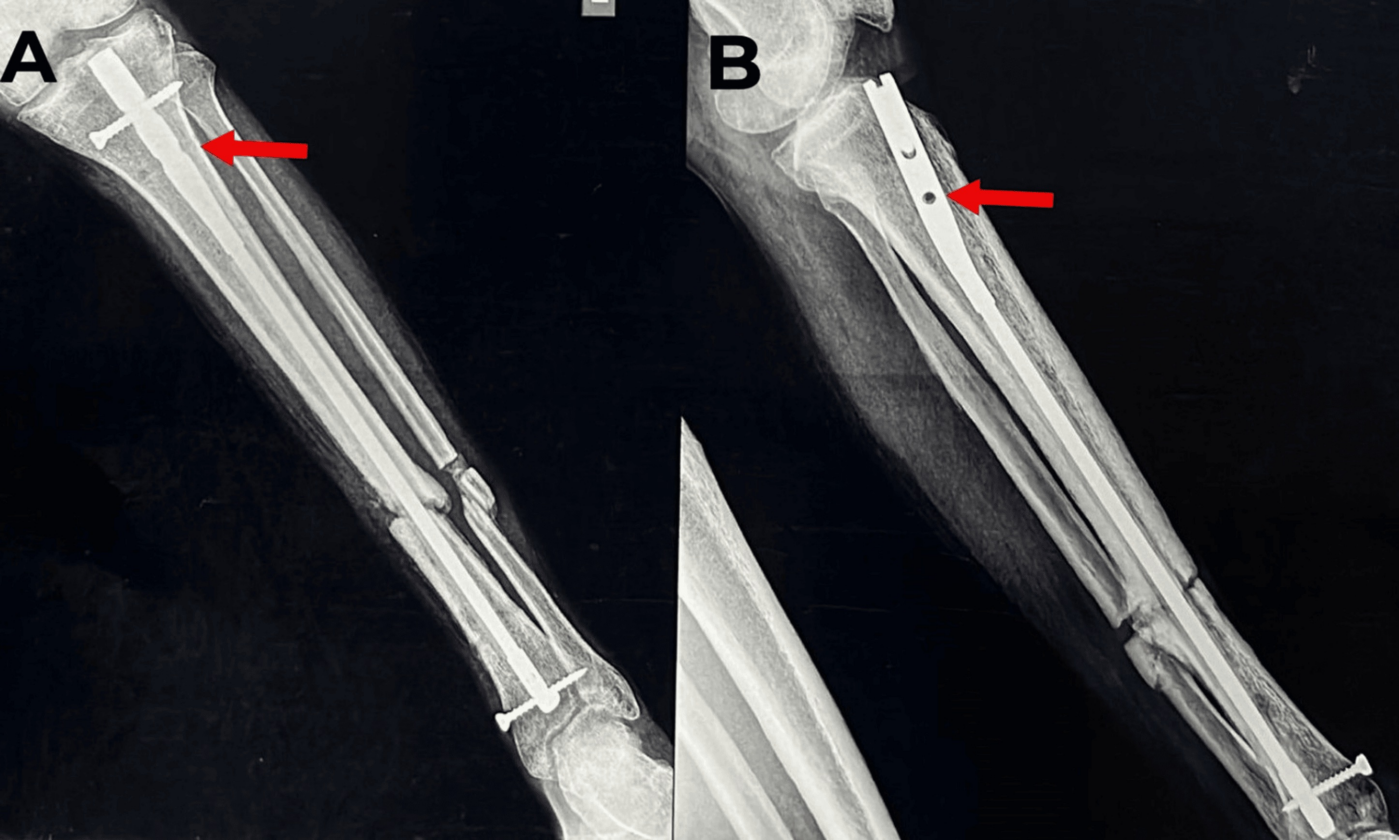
A-B) Left tibia and fibula AP and lateral X-ray views, respectively. Red arrows show dynamization. AP: anterior posterior
